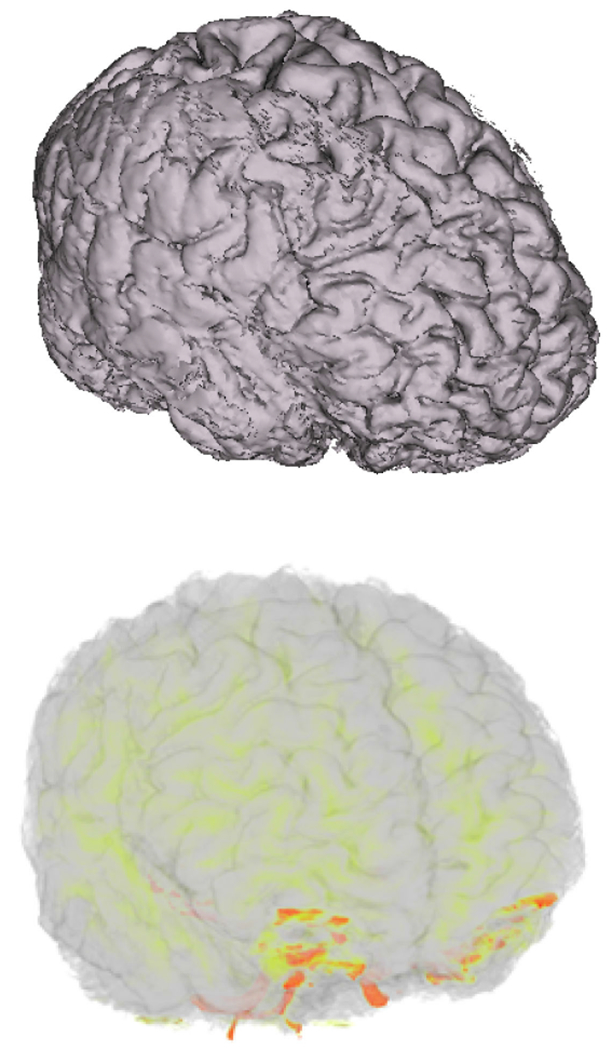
Human brain MRI dataset courtesy of the Mayo Clinic rendered in VisIt.
